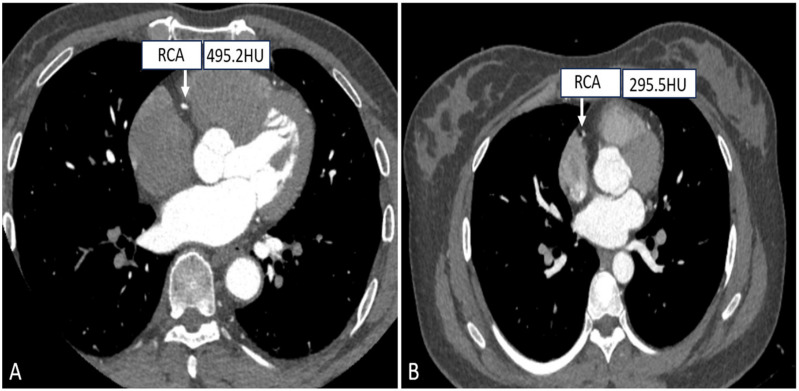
Right coronary CTA images with different SFOV. ((A) A patient with small SFOV; (B) A patient with large SFOV.).
